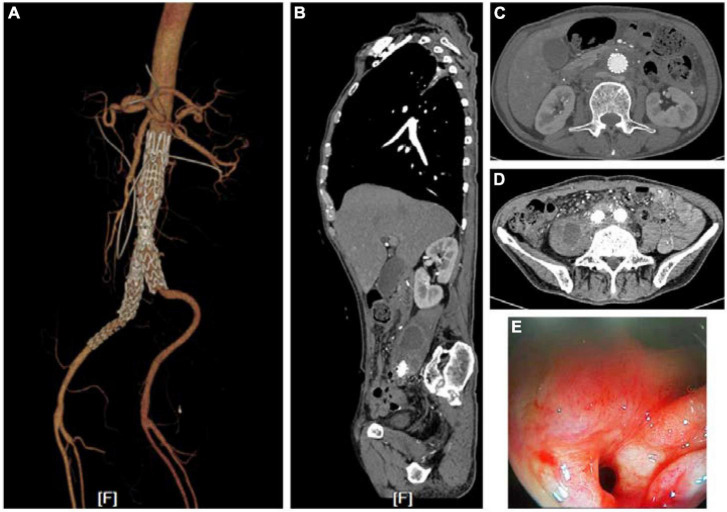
Images of CTA and endoscopy before treatments. (A) Endovascular repair and drainage had been performed when the patient was hospitalized. (B–D) A low-density shadow hinting at an abscess in the right psoas major muscle and around the stenting. (E) Fistulae in the horizontal part of duodenum found via endoscopy.
